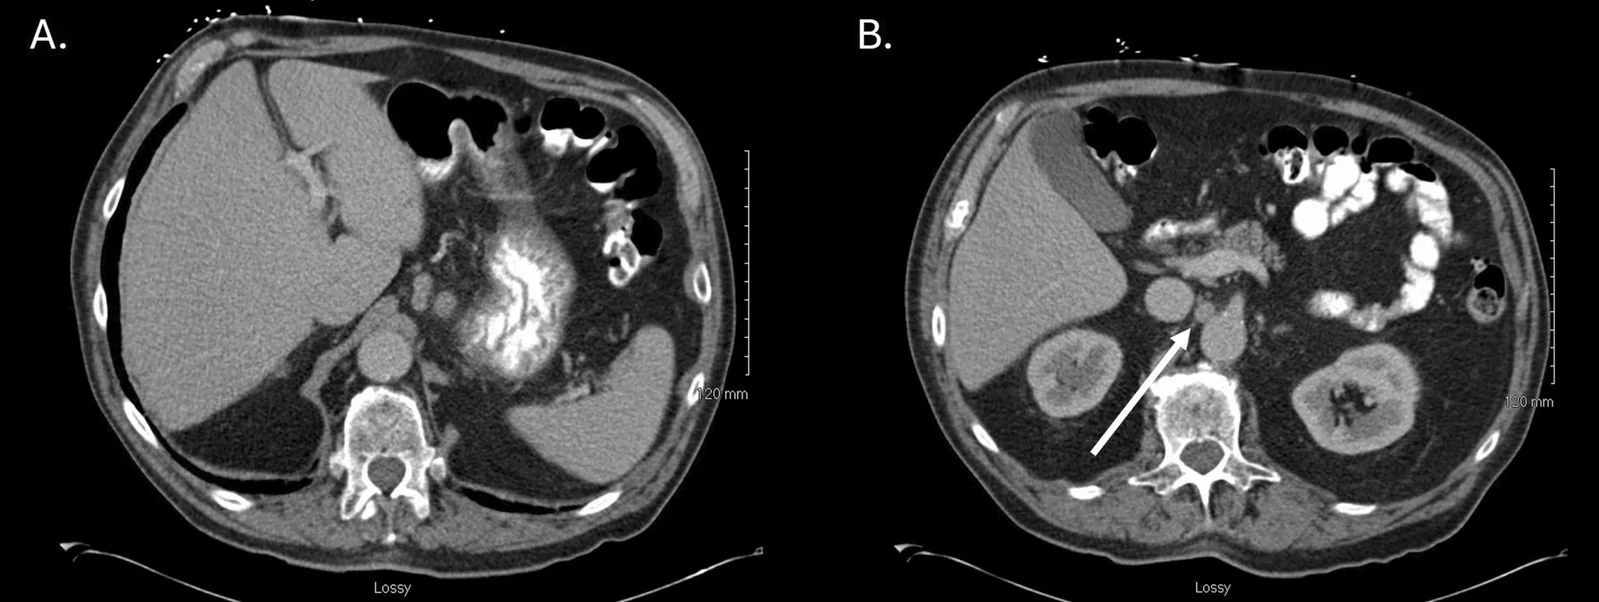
Baseline CT images depicting locally-advanced gastric cancer in an octogenarian with peri-gastric adenopathy (A) as well as an enlarged lymph node near the superior mesenteric artery (B, arrow)
